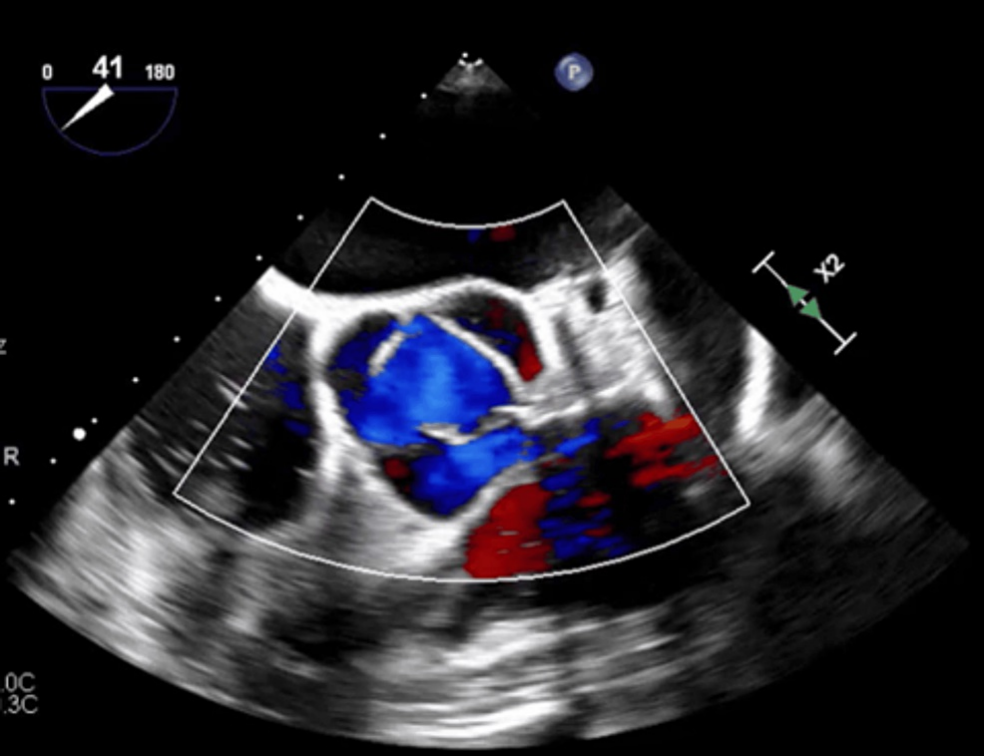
Post-procedural TEE showed a successfully ligated LAA with a very small residual scallop-shaped LAA with laminal flow. TEE: transesophageal echocardiogram; LAA: left atrial appendage
